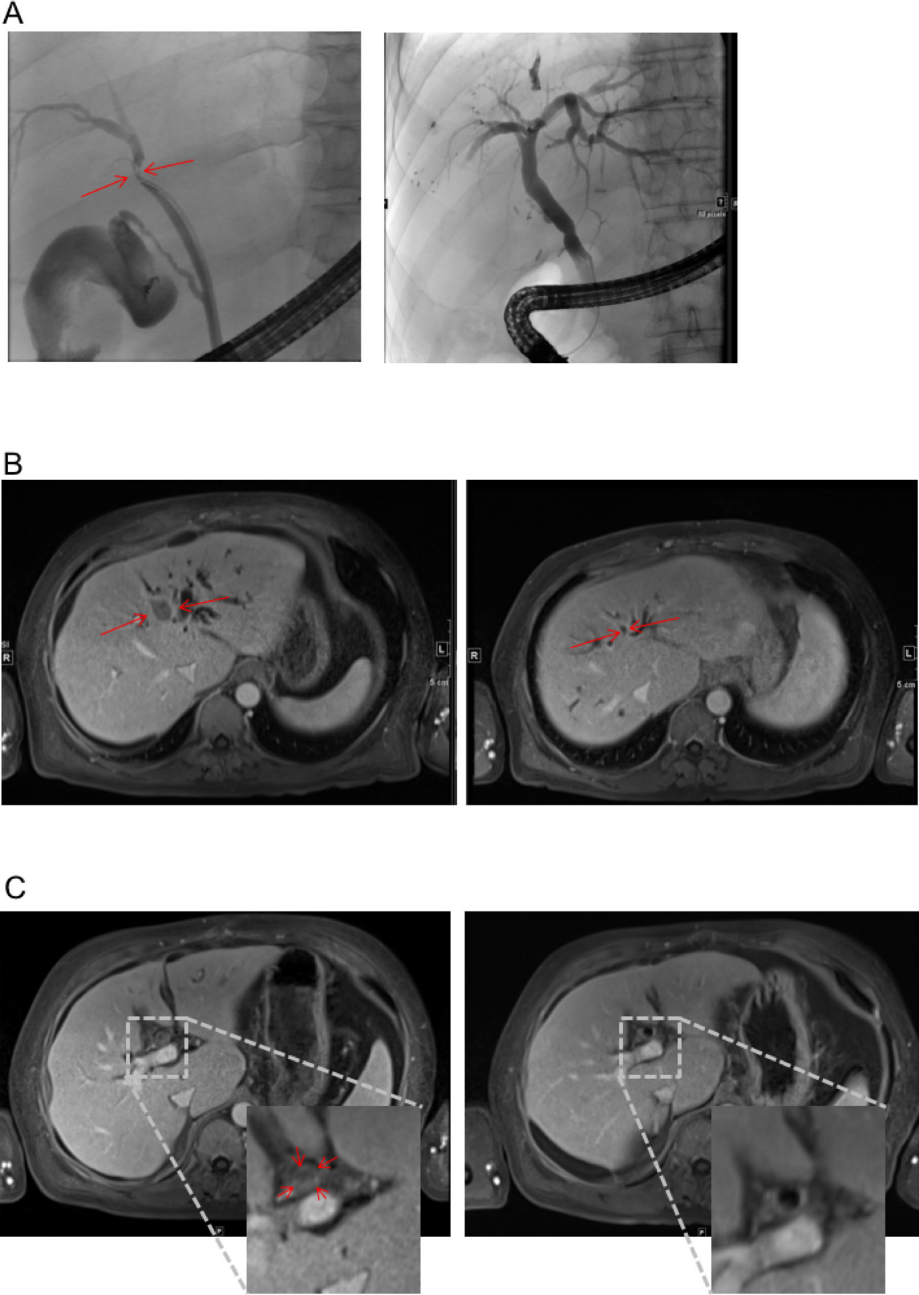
Response to therapy in MRI and ERC (A) ERC before placement of endoprothesis (left) and after successful therapy (right). Red Arrow heads indicate index lesion and relevant stenosis respectively. (B and C) MRI scan before (left) and after (right) 6 cycles of treatment according to FOLFOX6 regime. T1-weighted, Enlarged inset shows intraluminal tumor in the bile duct (C left).
